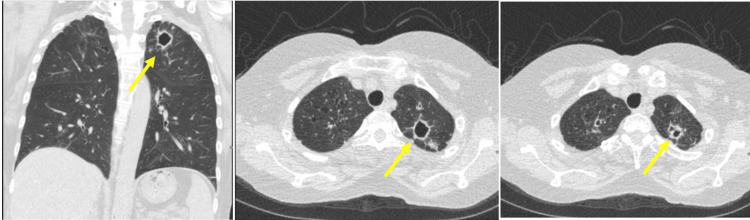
A lung CT scan showed multiple cavitary and solid nodules, including new ground glass opacities, observed bilaterally in lung apices with no mediastinal involvement (yellow arrow) CT: computed tomography
